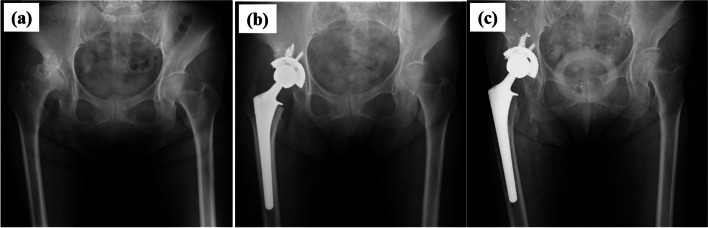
Pelvic radiographs. Radiograph of the pelvis (a) before right-side total hip arthroplasty, (b) at 2 weeks after right-side total hip arthroplasty, and (c) at 68 months after right-side total hip arthroplasty
